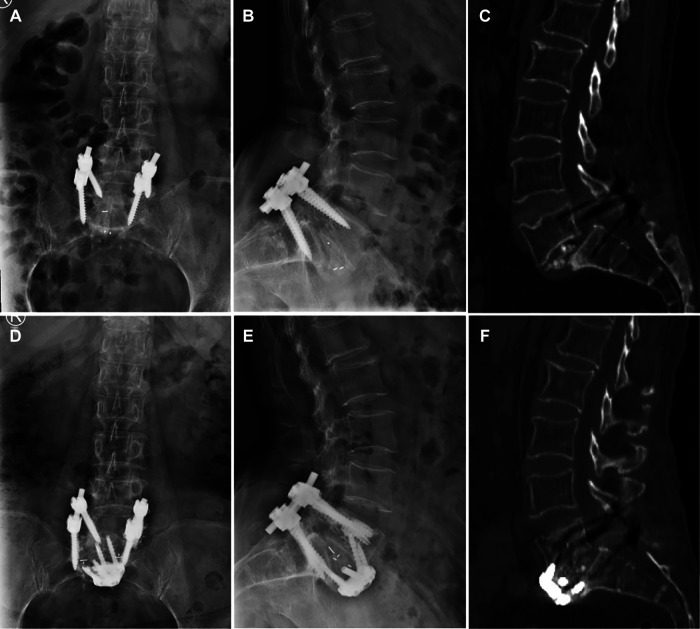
Preoperative frontal and lateral x-rays and CT, screw extraction, anterior slippage of a vertebral body, and cage displacement (A–C). Satisfactory repositioning of the vertebral body at 6 months postoperatively (D, E), with bone bridging seen on CT (F).
